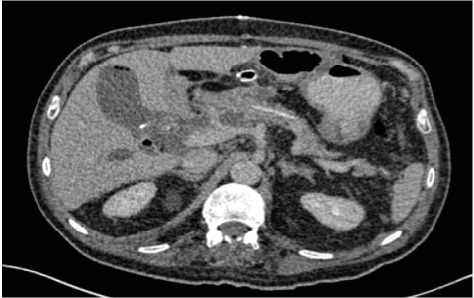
Postoperative CT demonstrating a single layer pancreaticojejunostomy using a dunking technique performed over a pancreatic duct stent.
